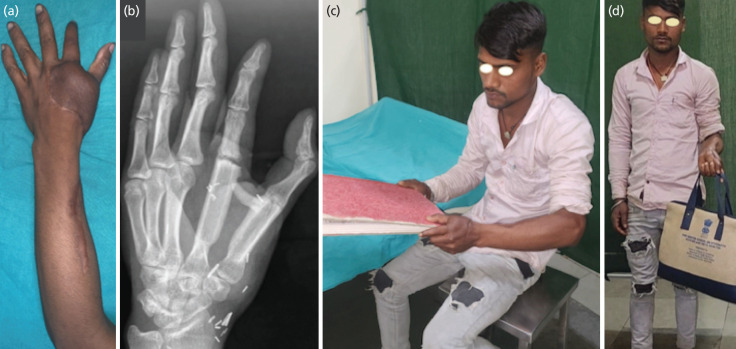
(a) Follow-up image showing a well-settled flap. (b) Follow-up radiograph showing fibular bone segments resembling metacarpals without bony resorption after six months. (c) Patient holding a book (Power grasp). (d) Patient lifting a bag (hook grip).
